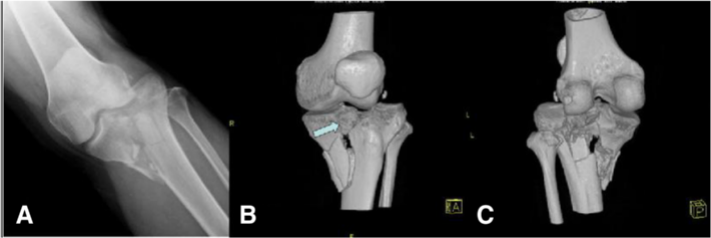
A patient with a Schatzker type V tibial plateau fracture from a motorcycle accident. a The plain radiograph shows a bicondylar fracture with a detached tibial eminence (insertion of anterior cruciate ligament [ACL]). b A three-dimensional (3D) reconstructed model of computed tomography (CT) scans shows a severely comminuted tibial eminence avulsion fracture (*arrow*) and a depression of the bicondylar articular surface. c The 3D model shows huge subchondral and metaphyseal bony defects
